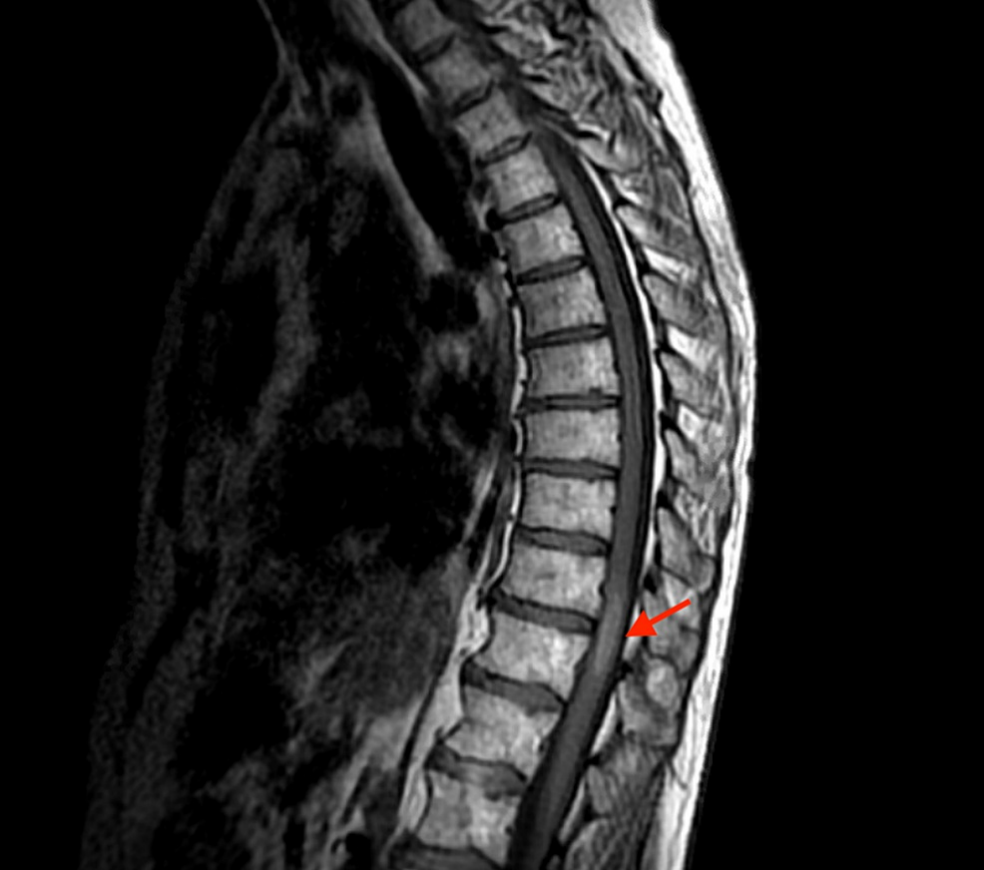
MRI of thoracic spine, T1 sagittal view. Minimal hyperintense T1 signal, suggesting intrinsic hemorrhage.
